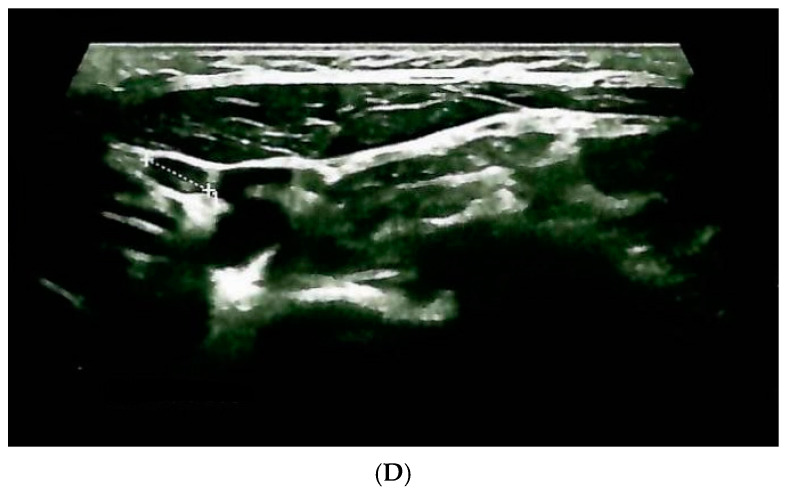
Thyroid ultrasound in a 6-year-old girl carrying *RET* pathogenic variant in codon 63. At the moment of ultrasound assessment, the patient presented high serum calcitonin levels. (A) Transversal plane. (B) Longitudinal plane of the right lobe. (C) Right lateral cervical adenopathy with maximum diameter of 1.1 cm. (D) Left lateral cervical adenopathy with nonspecific aspect and maximum diameter of 0.6 cm.
